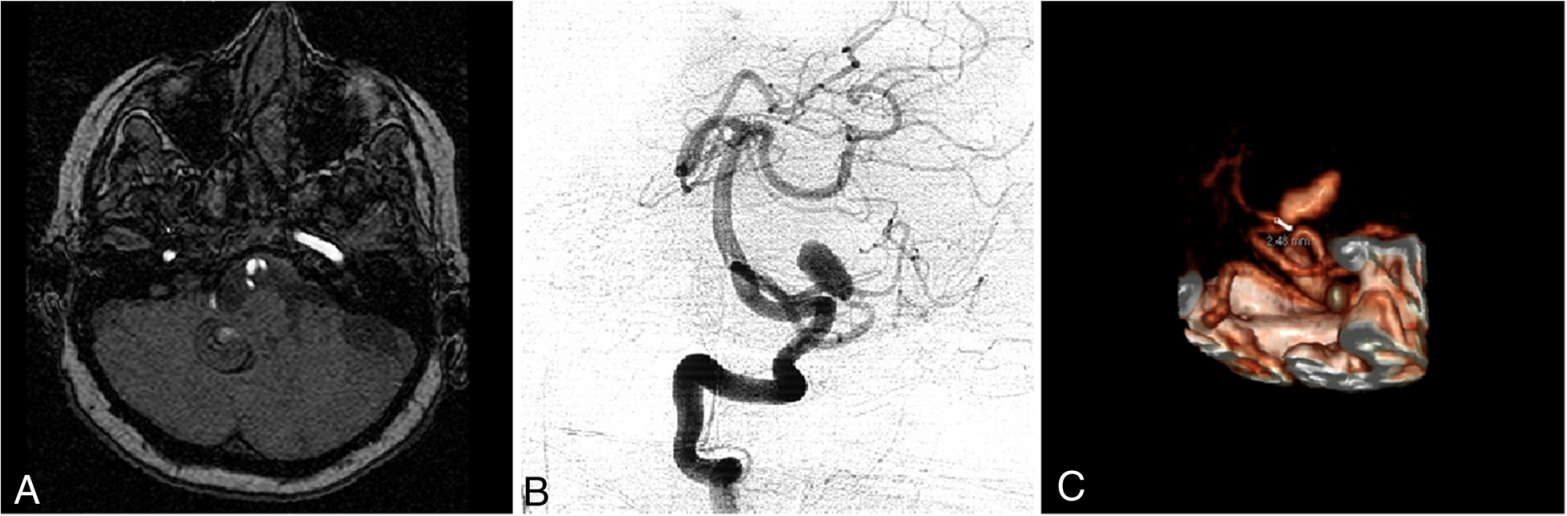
Pre-operative magnetic resonance imaging studies. (A) Post-contrast-enhanced T1-weighted magnetic resonance angiogram. (B) Digital subtraction angiography showing lateral view. (C) Three-dimensional reconstructed image showing the neck of the aneurysm.
